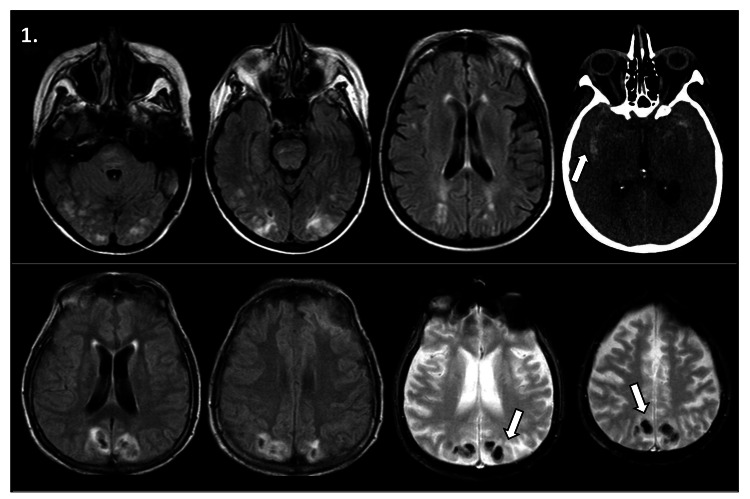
Hemorrhages in the context of reversible posterior leukoencephalopathy syndrome Two different patients, one in the upper panel and another in the lower panel (Upper panel, center and left images) Axial brain magnetic resonance imaging (MRI) in FLAIR sequence, showing bioccipital cortico-subcortical and brainstem hyperintense areas consistent with vasogenic edema. (Upper panel, far right image) Axial brain computed tomography (CT) 48 hours later, demonstrating hyperdense areas in both Sylvian fissures compatible with subarachnoid hemorrhage. (Lower panel, center and left images) Brain MRI in FLAIR sequence displaying bioccipital cortico-subcortical hyperintense areas. (Lower panel, center and right images) Brain MRI in GRE sequence exhibiting concentric hypodense areas in the bioccipital region consistent with intraparenchymal hematoma.
